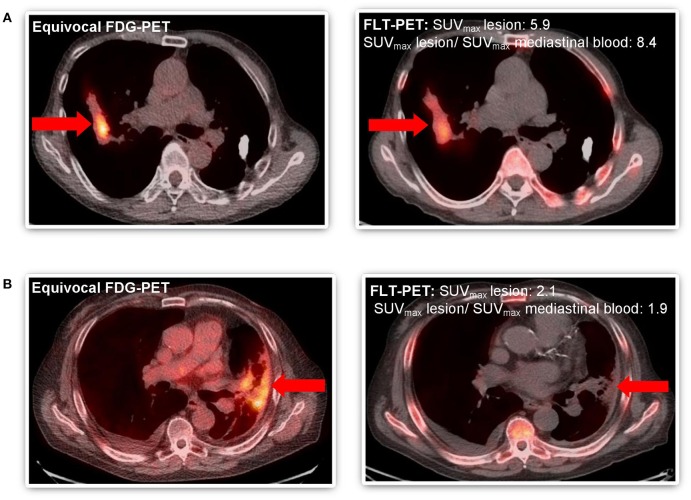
Comparative imaging of FDG-PET and FLT-PET in patients with equivocal follow-up FDG-PET scan after SABR to thoracic malignancy. (A) Patient #4 WITH disease recurrence predicted by FLT-PET and confirmed by progression on subsequent serial imaging (both SUV_max_lesion >2.0 and ratio SUV_max_ lesion and mediastinal blood pool >2.0) and focal FLT uptake. (B) Patient #8 WITHOUT disease recurrence predicted by FLT-PET and confirmed by biopsy (SUV_max_lesion >2.0 but ratio SUV_max_ lesion and mediastinal blood pool < 2.0). Equivocal lesion by FDG-PET highlighted by arrow (
